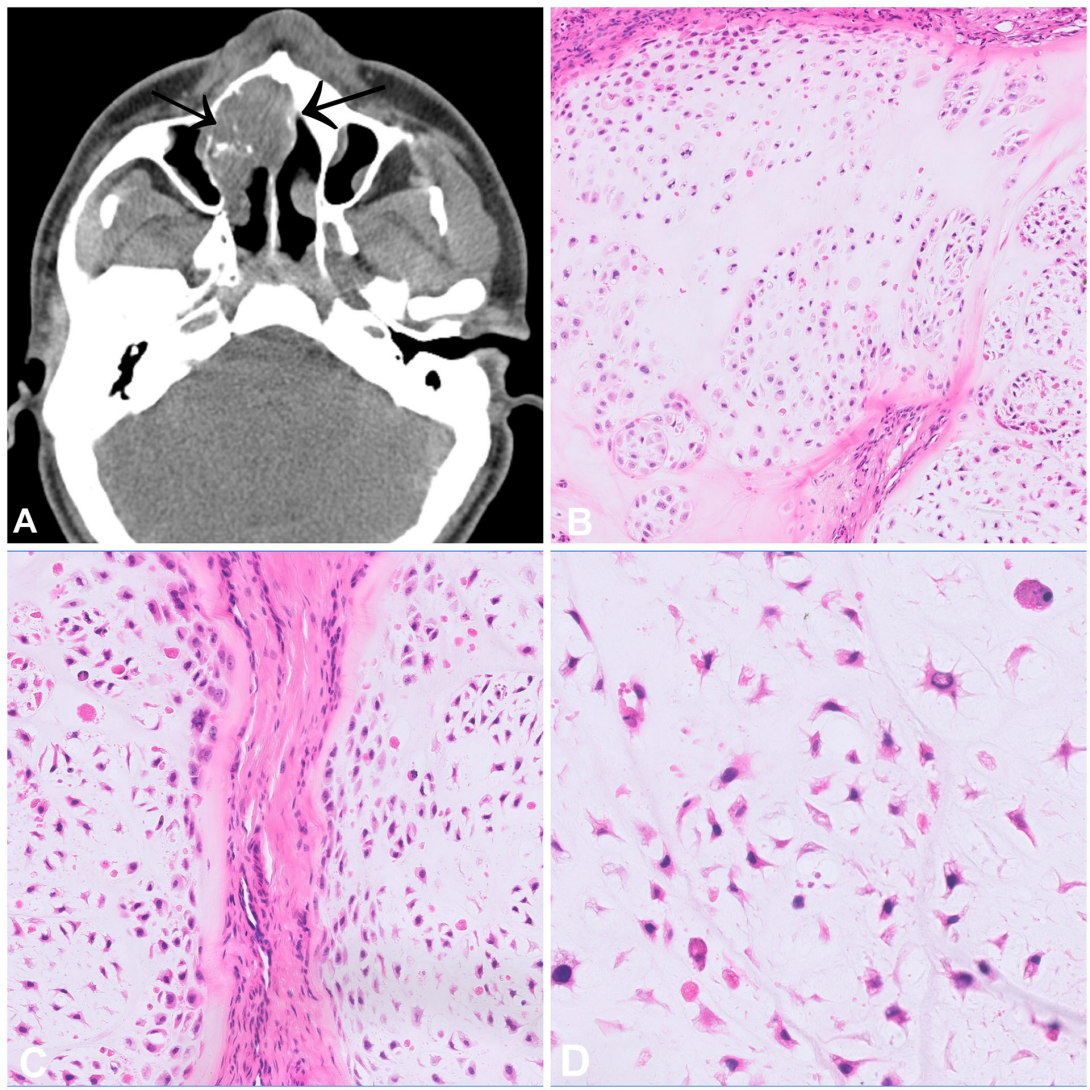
Chondrosarcoma. (A) CT scan of a high-grade chondrosarcoma arising from the nasal septum with focal mineralisation. (B–D) Grade 2 chondrosarcoma showing increase in cellularity when compared to a chondrosarcoma grade 1. The cells have atypical, hyperchromatic nuclei and are set in a hyaline matrix with myxoid change.
